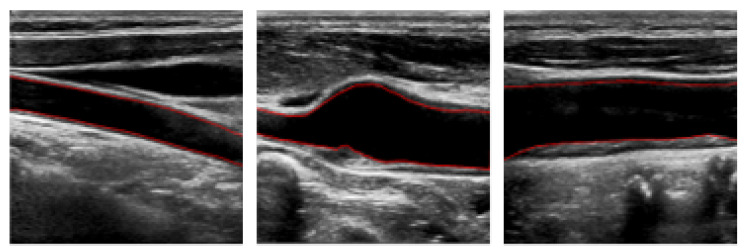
Ultrasound images of carotid artery. The red lines represent the lumen walls.
